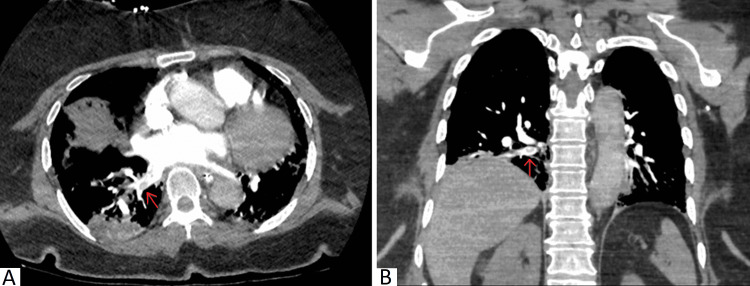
Axial (A) and coronal (B) images on CT angiography demonstrating a filling defect in the right lower lobe pulmonary vein, consistent with pulmonary vein thrombosis.
